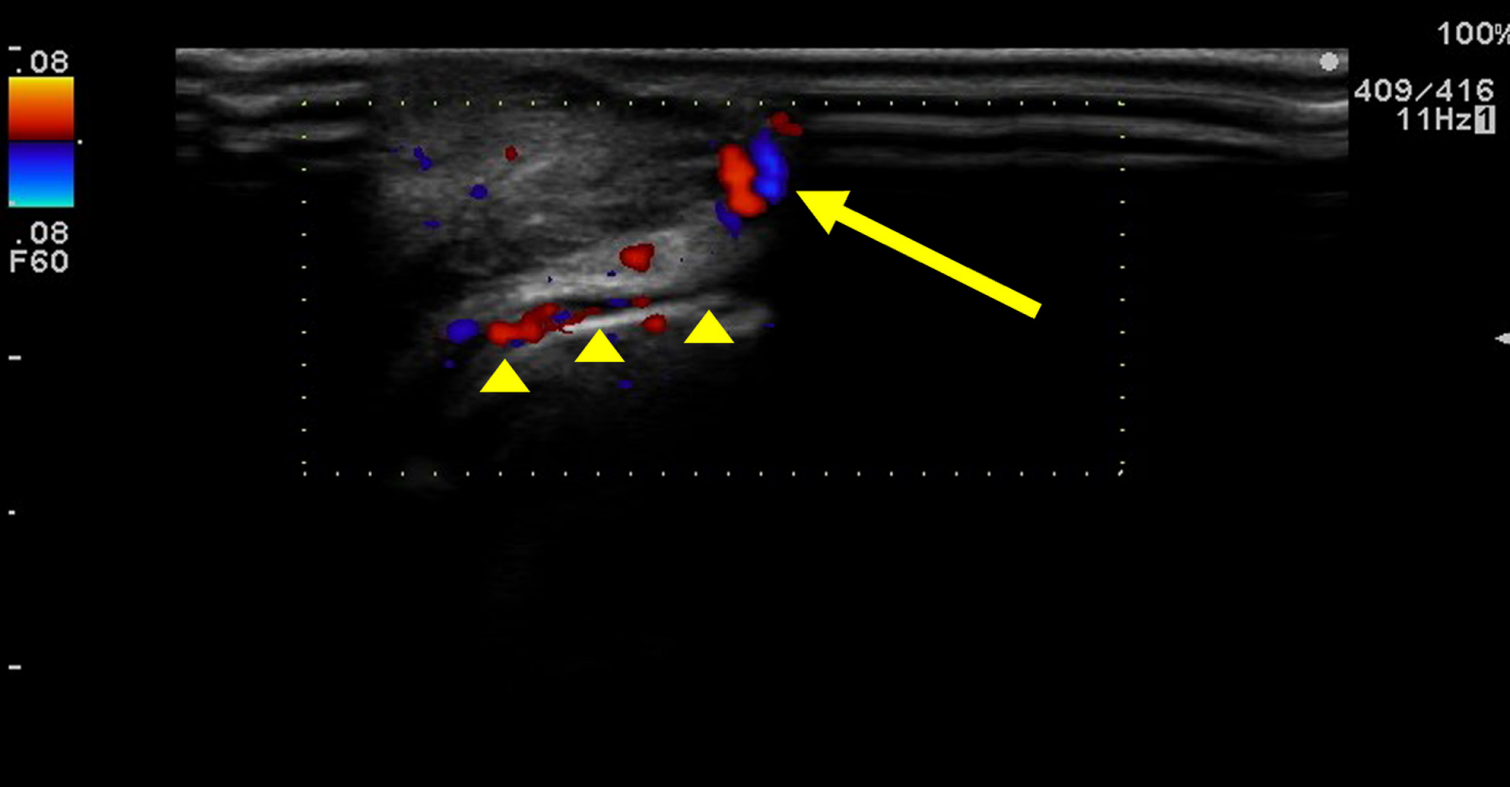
A mucosal perforator arising from a blood vessel running through the orbicularis oris muscle was identified on the center of the lower red lip and marked using a color Doppler ultrasound. Arrowheads indicate the blood vessel running through the orbicularis oris muscle. The arrow indicates the mucosal perforator rising from the blood vessel.
